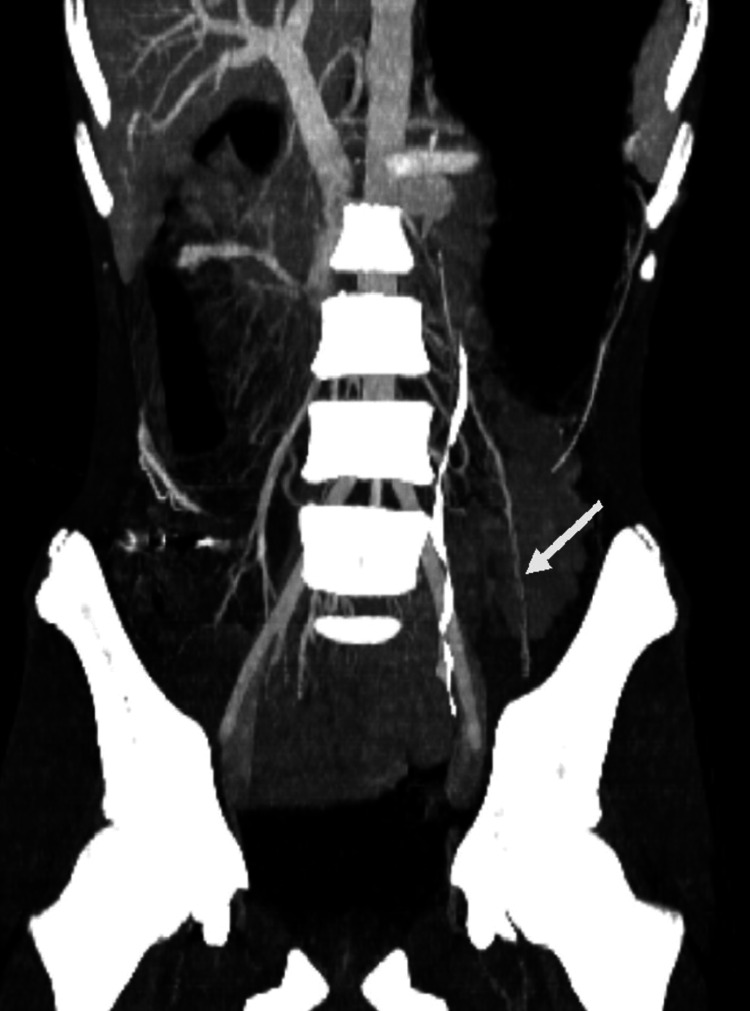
Contrast-enhanced computed tomography (CT); coronal reconstruction in the portal venous phase shows dilation of the left gonadal vein (white arrow).
